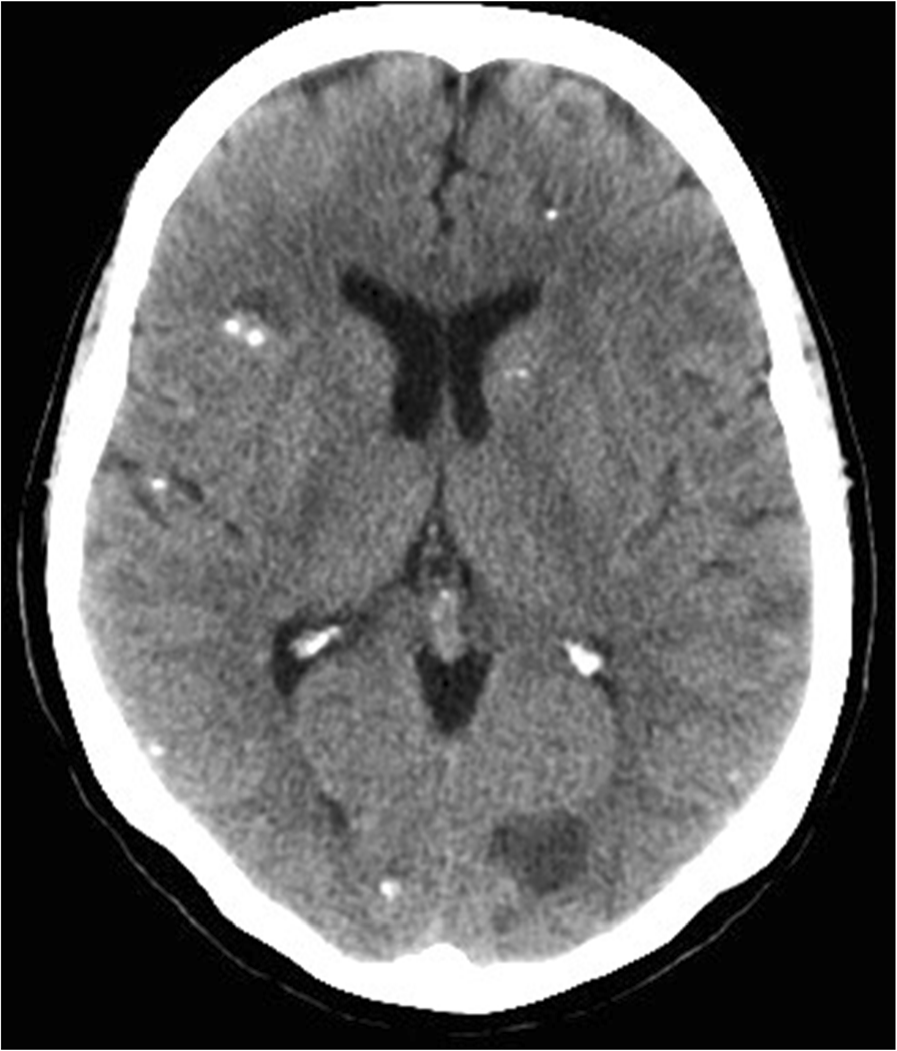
Computed tomography of the head demonstrated multiple bilateral well-defined cystic structures of different sizes associated with calcification without perilesional edema
